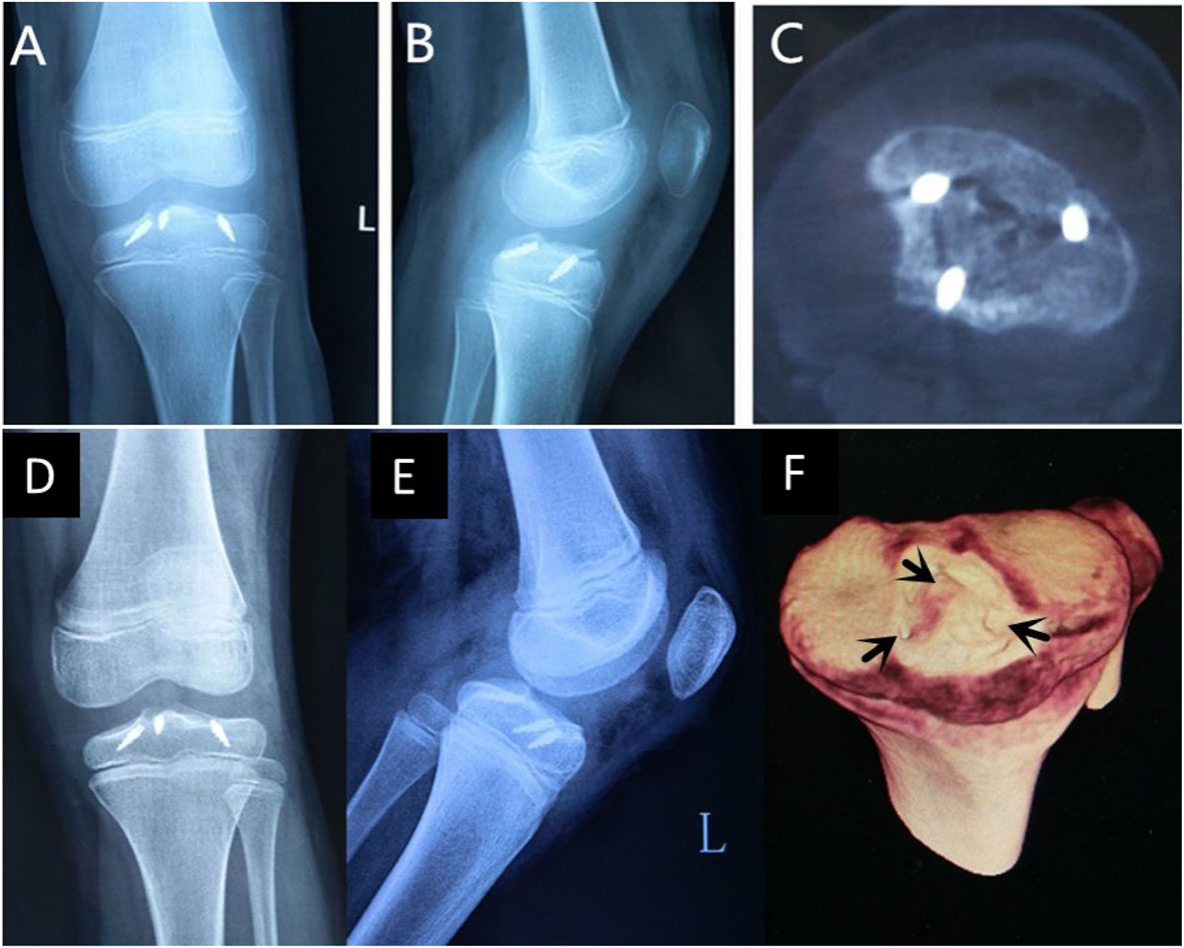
Post-operation images. a: Conventional radiography anterior posterior (AP) and b: lateral view and c: computed tomography (CT) scan immediately after surgery. d: Conventional radiography AP and e: lateral view and f: CT scan 6-months post-operation. Showing good epiphyseal union of the fracture
